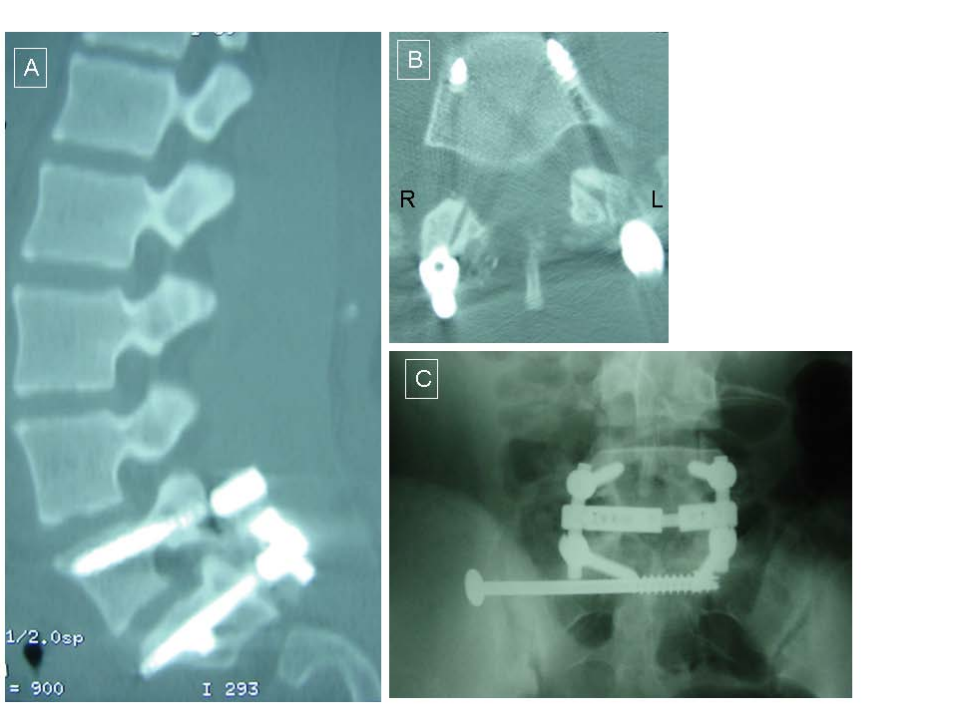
Post-operative images. Panel a) Sagittal CT reconstruction following reduction of the anterolisthesis of L5 on S1 with posterior instrumentation. Axial CT scan in Panel b) shows the pedicular screws of L5 with anatomical reduction of the left locked L5-S1 facet joint. In Panel c), AP X-ray of the lumbosacral area with reduced right sacroiliac joint following SI joint screw fixation.
